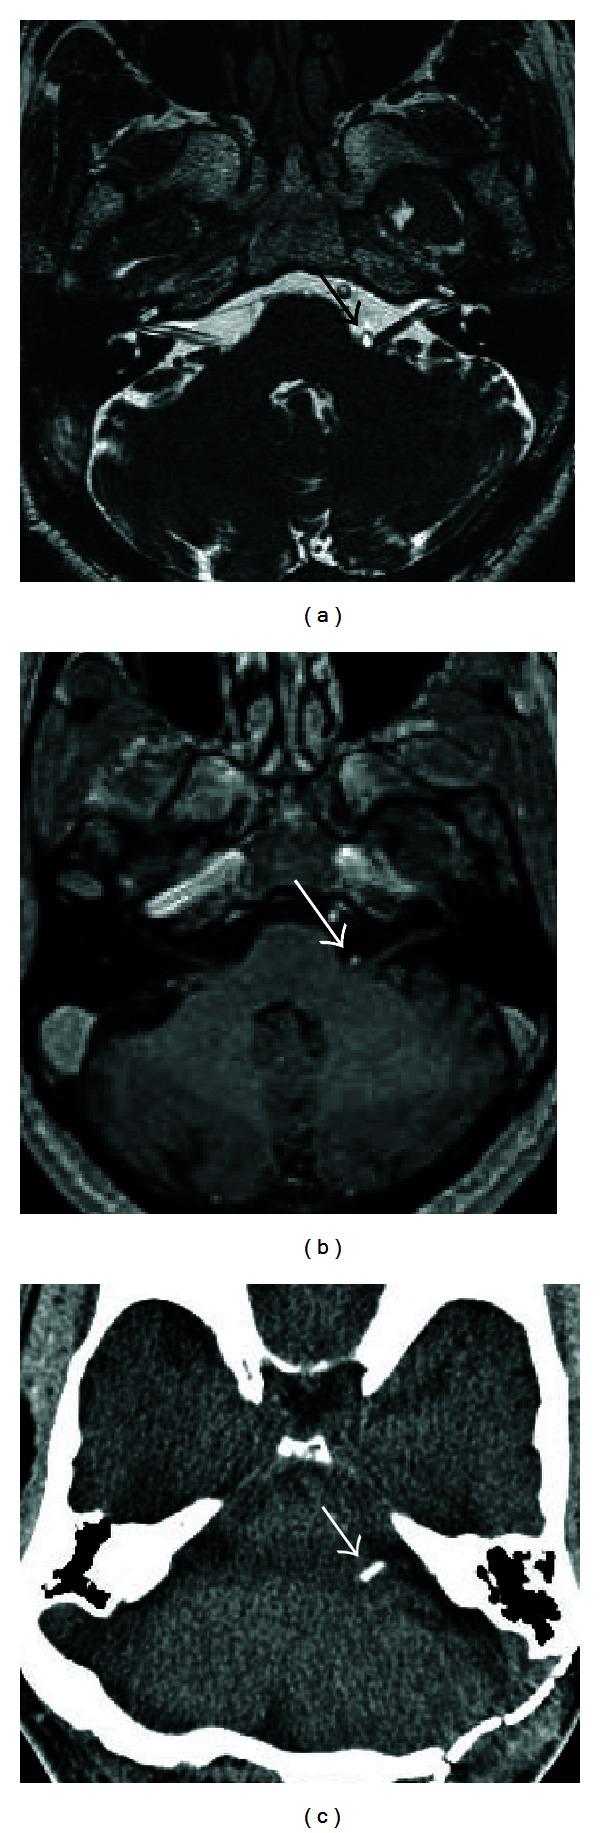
Neurovascular compression in a patient presenting with left hemifacial spasm. Axial CISS (a) and T1 postcontrast MRI (b) images demonstrate the left vertebral artery (arrow) abutting the left facial nerve at the root entry zone. Axial noncontrast CT scan (c) demonstrates the hyperdense postsurgical material from microvascular decompression surgery.
